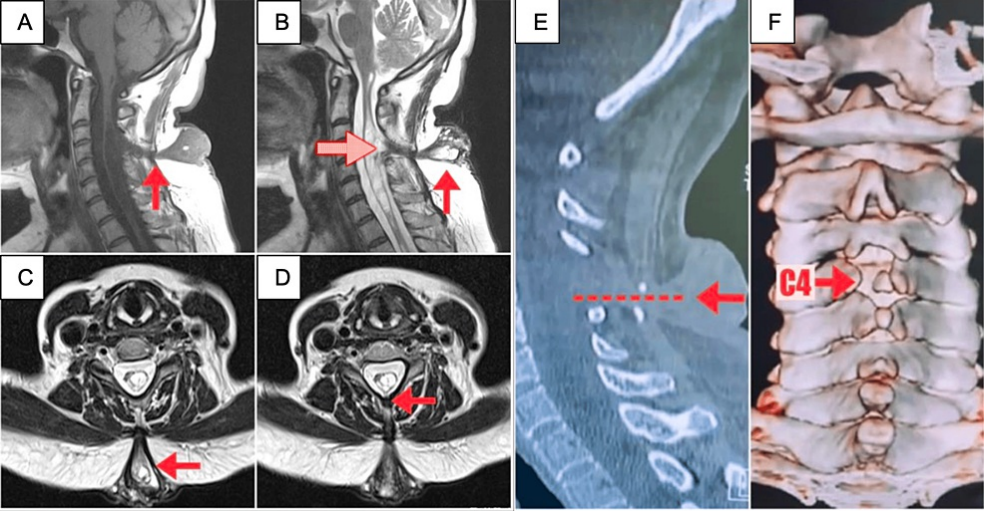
Preoperative imaging (A-F) MRI and CT scan during diagnostic assessment. Sagittal T1 and T2 (A-B) images show the crucial path of the lesion (red arrow) identified from the skin to the spinal cord. Note the dermal sinus extending cranially from the opening lesion. Tethering causes a dorsal displacement of the cord and syringomyelia (dotted red arrow). (C-D) Axial T2 imaging demonstrates the tract crossing all skin layers heading to the spinal cord (red arrows). CT scan (E-F) displayed the conduit trajectory in the sagittal bone window (arrow and dotted line). Coronal CT scan with three-dimensional reconstruction (F); posterior view showing dysraphism of the spinous process and posterior arch of C4 (red arrow). C3 and C5 exhibit dysraphism with small spinous processes and notches allowing abnormal tract continuity.
